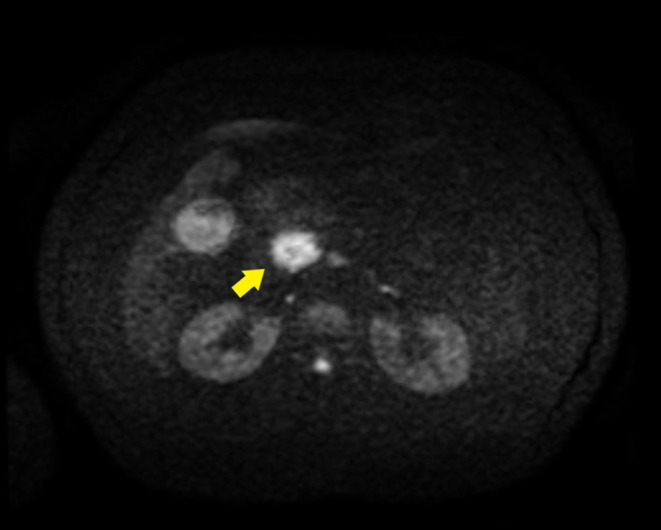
MRI showed a mass‐like lesion around the head of the pancreas that was hyperintense on diffusion‐weighted imaging(arrow). MRI, magnetic resonance imaging.
